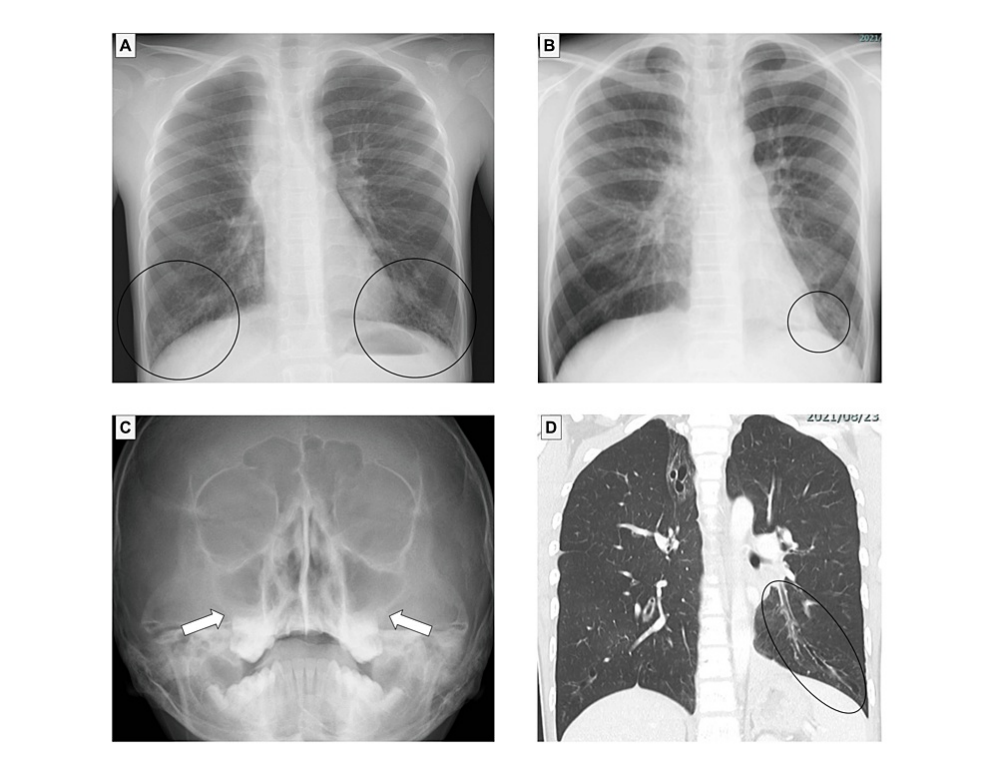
Imaging findings in Case 2. (A) Chest X-ray at 5 years of age showed infiltrative shadows (circles) in the bilateral lower lung. (B) Chest X-ray showed atelectasis in the left lower lobe (circle). (C) Nasal X-ray at 13 years of age showed thickened mucosa of the bilateral maxillary sinuses (arrows). (D) Chest computed tomography at 13 years of age showed bronchiectasis (circle).
